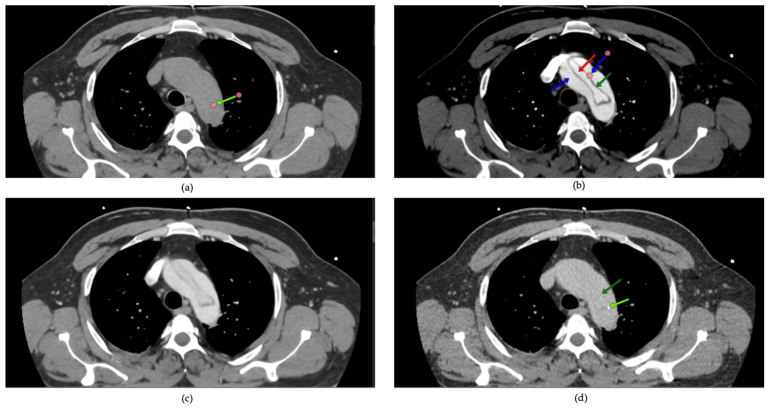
Multiphase CT imaging of Stanford Type A aortic dissection: true and false lumen analysis; (a–d): axial CT images demonstrating characteristic findings of Stanford type A aortic dissection. Dark green arrow: intimal flap, marking the division between the true and false lumen; red arrow: true aortic lumen, compressed and reduced in size due to the higher pressure within the false lumen; blue arrow: false aortic lumen, which surrounds the true lumen and shows delayed opacification (arterial phase visible (b) versus venous phase in (c)); light green arrow: intimal calcifications within the aortic wall.
